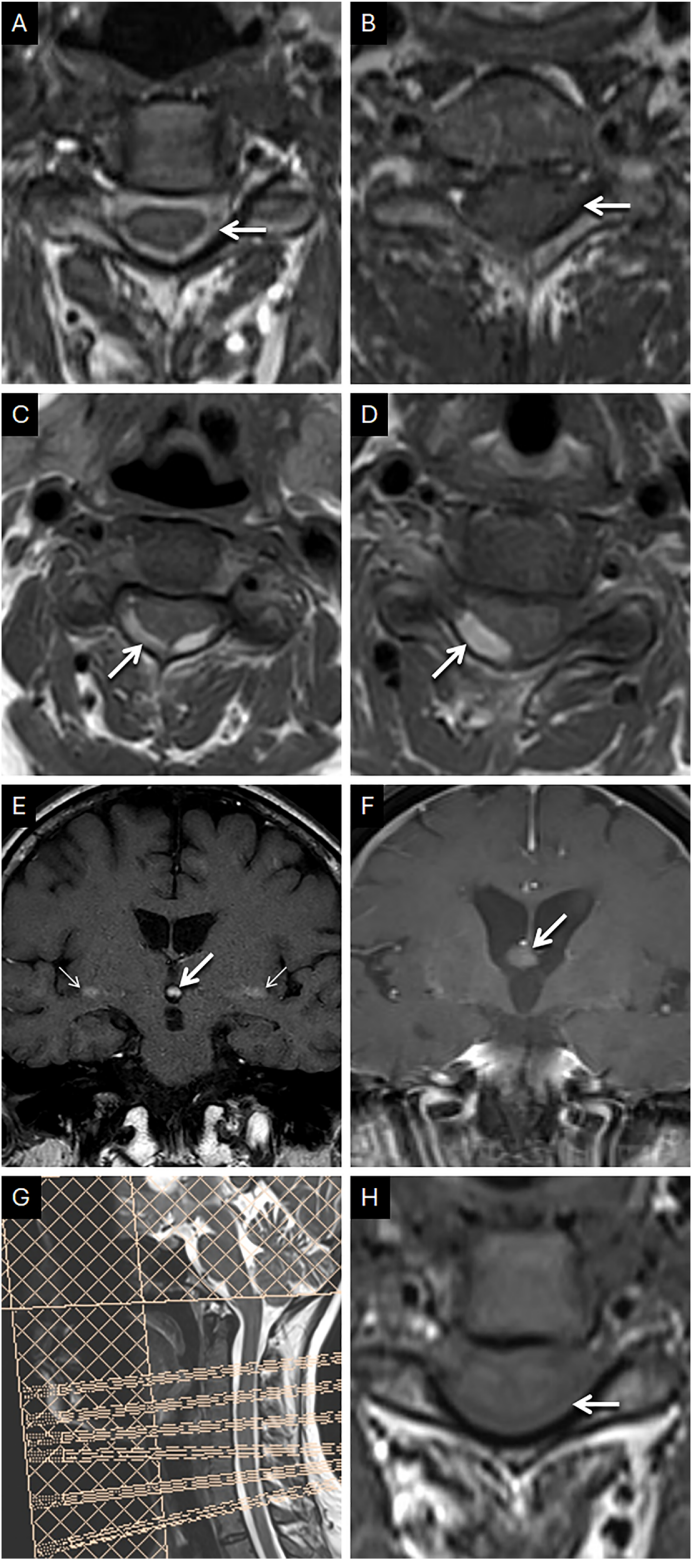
Usual appearances of ESP and its potential pitfalls along with the method of rectification. Typical appearances of ESP (A, B). Axial sequential craniocaudal T1 images (A, B) through the cervical spine, in a 35-year-old presenting with cervicogenic headache, reveal hyperintense signals within the thecal sac in the upper cervical spine (arrow in A) with progressive signal loss caudally (arrow in B). Extra-axial hemorrhage mimicking ESP (C, D). Axial sequential craniocaudal T1 images (C, D) through the cervical spine, in a 20-year-old presenting with polytrauma, reveal hyperintense signals (arrows) along the cervical cord representing subdural hemorrhage. Note the lack of progressive fading of the intensity (compared to flowing CSF in A, B). Pitfalls in interpreting ESP (E, F). Coronal T1 image (E) in a 16-year-old presenting with precocious puberty reveals a focus of hyperintensity (arrow) within the third ventricle related to ESP. This artifactual signal faded out on deeper coronal slices (not shown). Note the phase encoding artifacts (thin arrows) seen in the adjacent brain parenchyma, in linear relation with the artifactual bright signal within the third ventricle, confirming CSF flow. Coronal contrast-enhanced T1 image (F) in a 50-year-old patient presenting with headache reveals an incidental, round, relatively hyperintense mass lesion along the roof of the third ventricle in keeping with a colloid cyst (arrow). It is important to consider the site of the lesion and the absence of adjacent phase encoding artifacts (which are only associated with mobile spins). Method of rectification of ESP (G, H) in a 40-year-old presenting with bilateral upper limb radiculopathy. Sagittal scout image (G) obtained for planning of a cervical spine MRI with additional saturation band across the posterior fossa to null ESP from the craniocaudal flow of CSF. Axial T1 image (H) acquired subsequently completely saturates CSF signals in the thecal sac (arrow)
